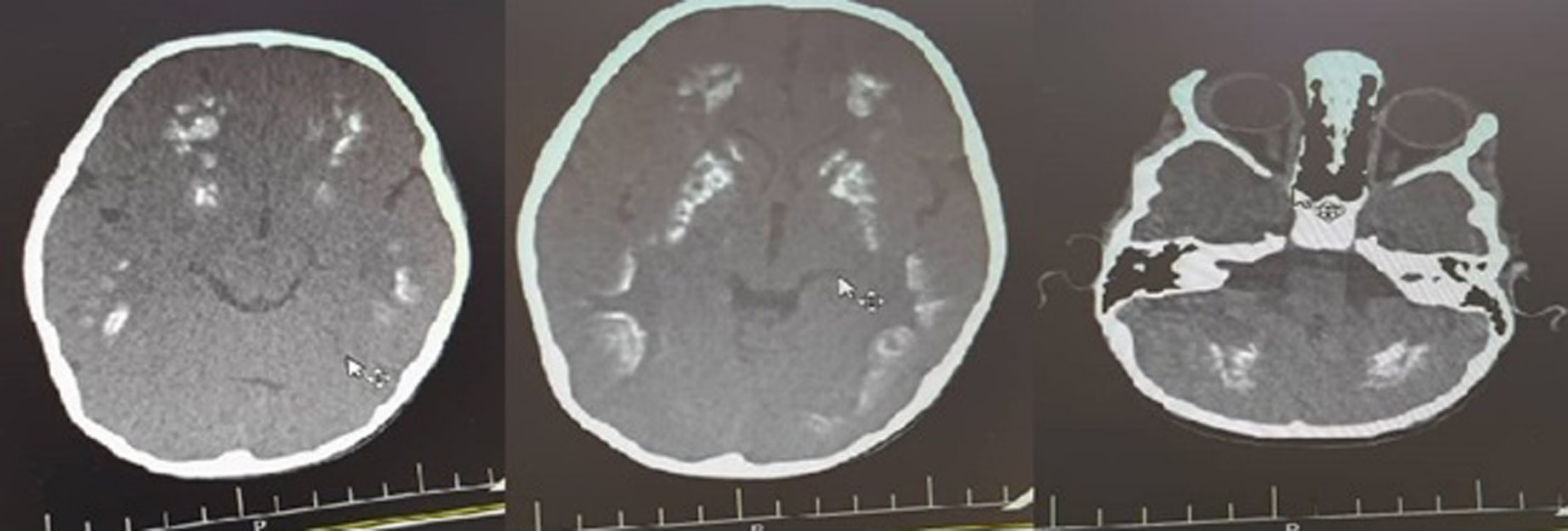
Axial planes of brain CT‐scan of the younger sibling demonstrate diffuse symmetrical calcifications
